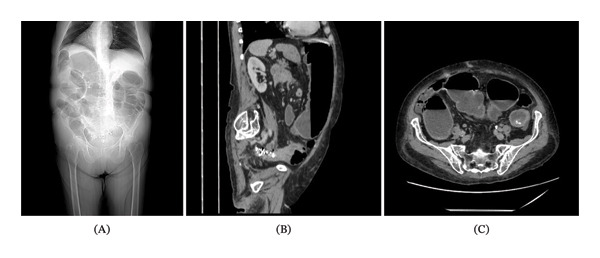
Abdominal CT: intestinal distention (A), obstruction of colonic transit in the sigmoid colon with residual contrast (B), and colonic distension with air–fluid levels (C).
